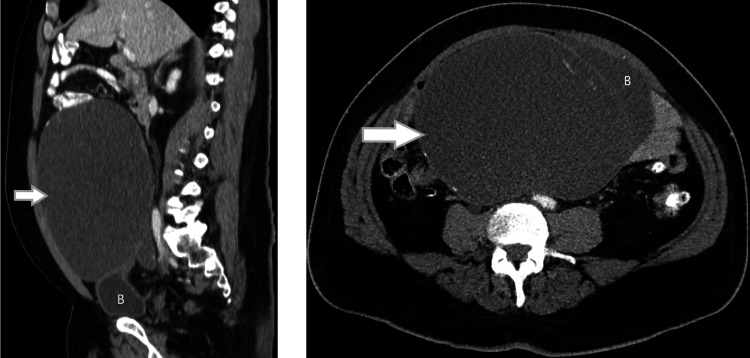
Sagittal and axial image from contrast enhanced CT demonstrating a cystic tumor (see arrow) arising from the dome of the urinary bladder (labeled “B”) corresponding to a urachal cystic tumor of low malignant potential.
